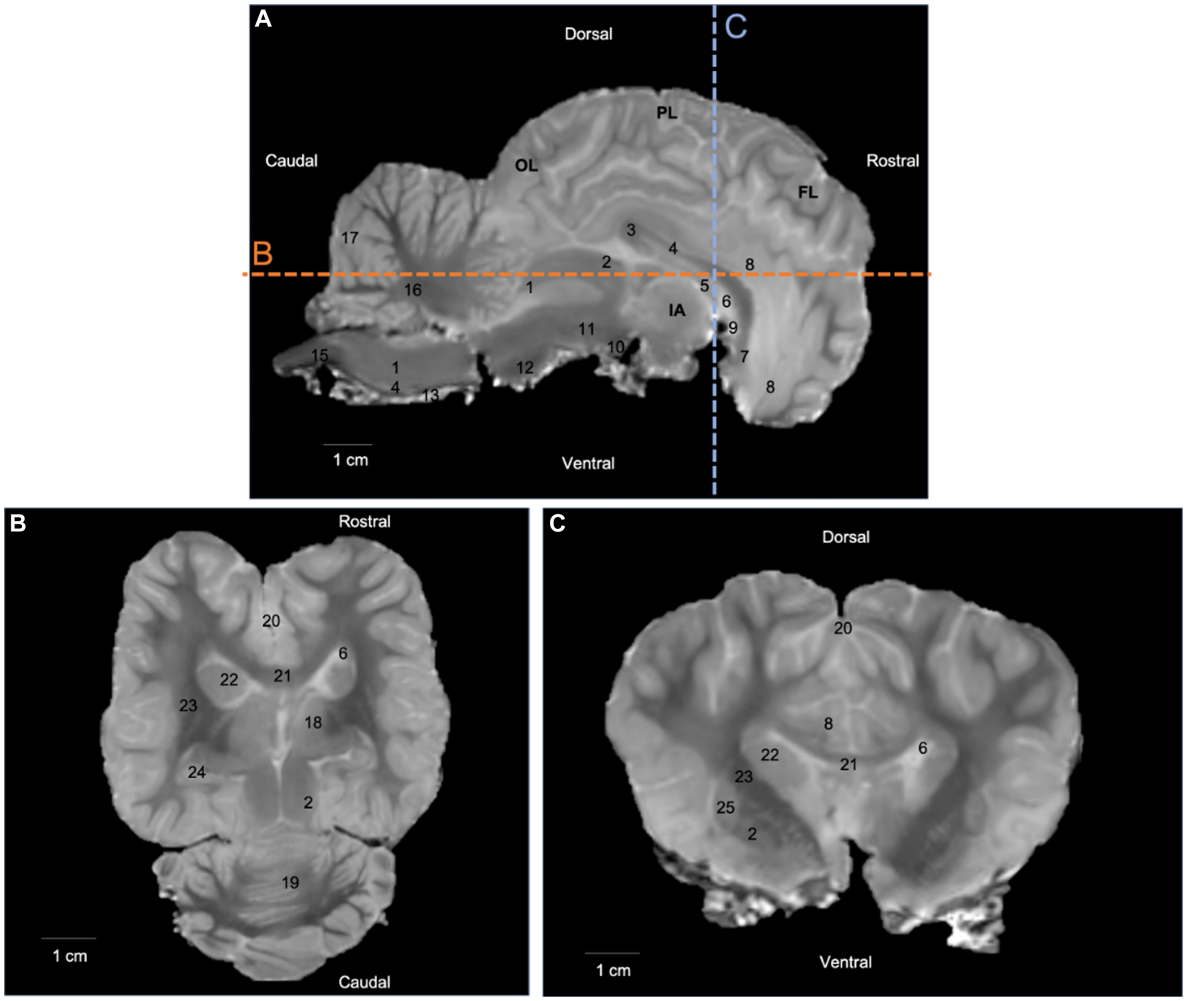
T2-weighted images in (A) median plane, (B) dorsal plane, and (C) transversal plane. Lines b and c on the median plane correspond to the section planes. OL: occipital lobe; PL: parietal lobe; FL: frontal lobe; IA: interthalamic adhesion; 1: caudal colliculus; 2: rostral colliculus; 3: corpus callosum (splenium); 4: corpus callosum (body); 5: fornix; 6: lateral ventricle; 7: corpus callosum (genu); 8: cingulate gyrus; 9: rostral commissure; 10: mammillary body; 11: cerebral peduncle; 12: pons; 13: pyramidal tract; 14: medulla oblongata; 15: spinal cord; 16: cerebellar medulla; 17: cerebellar cortex; 18: thalamus; 19: cerebellum (vermis); 20: longitudinal fissure; 21: corpus callosum; 22: caudate nucleus; 23: internal capsule; 24: hippocampus; 25: putamen; 26: globus pallidus.
